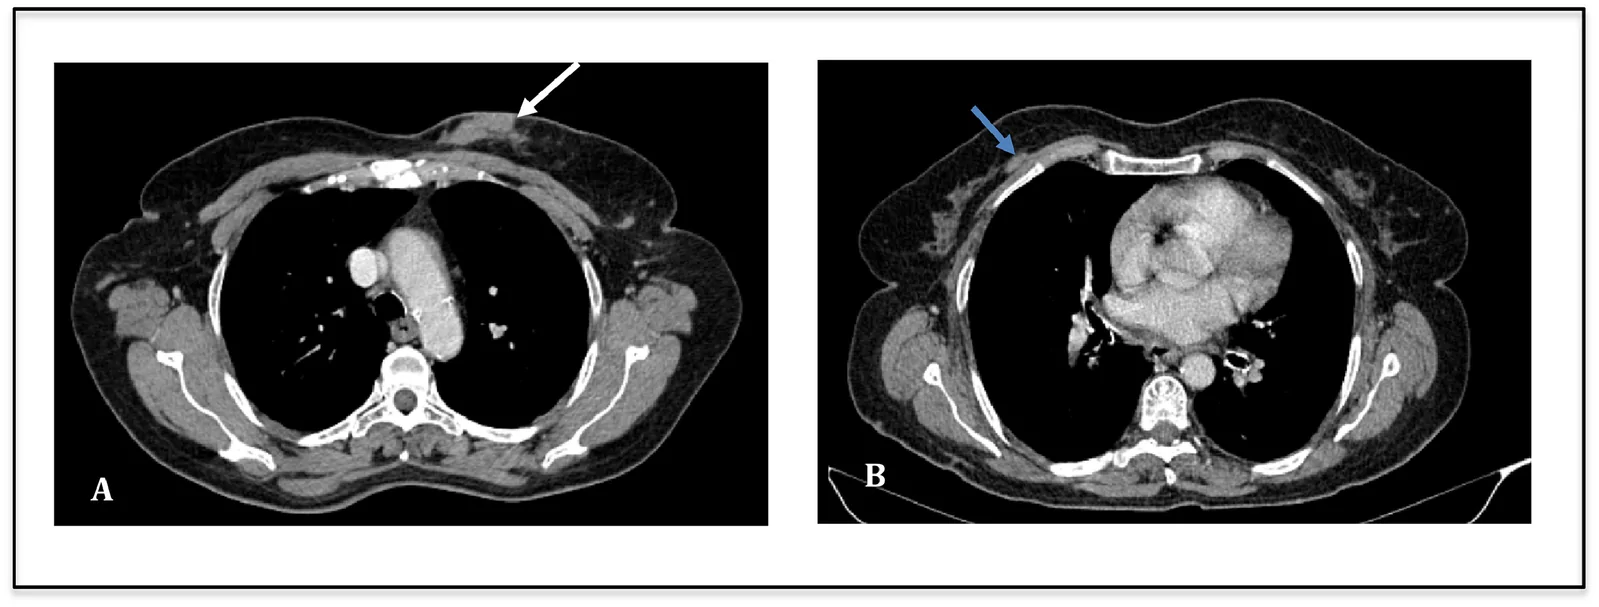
Computed tomography revealed bilateral breast masses, confluent and oblong in shape, with well-defined regular contours. (A) upper inner quadrant of the left breast (arrow white); (B) lower inner quadrant of the right breast (arrow blue).
